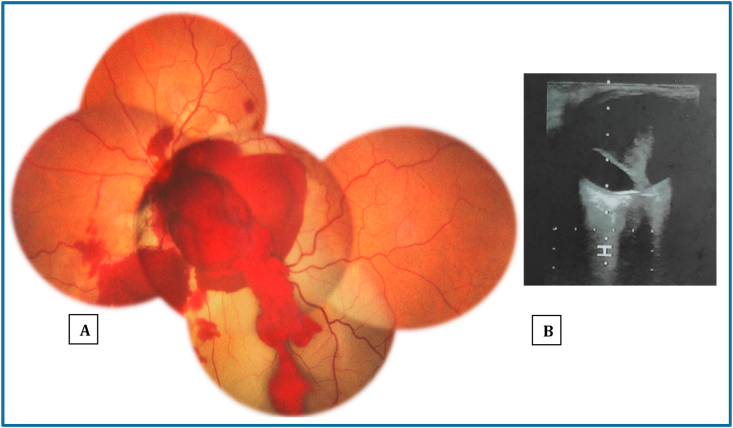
Initial examination. A: Fundus photography reveals an extensive vitreous and preretinal hemorrhage overlaying the optic disc and retina edema. B: Ocular ultrasound shows posterior vitreous detachment.
